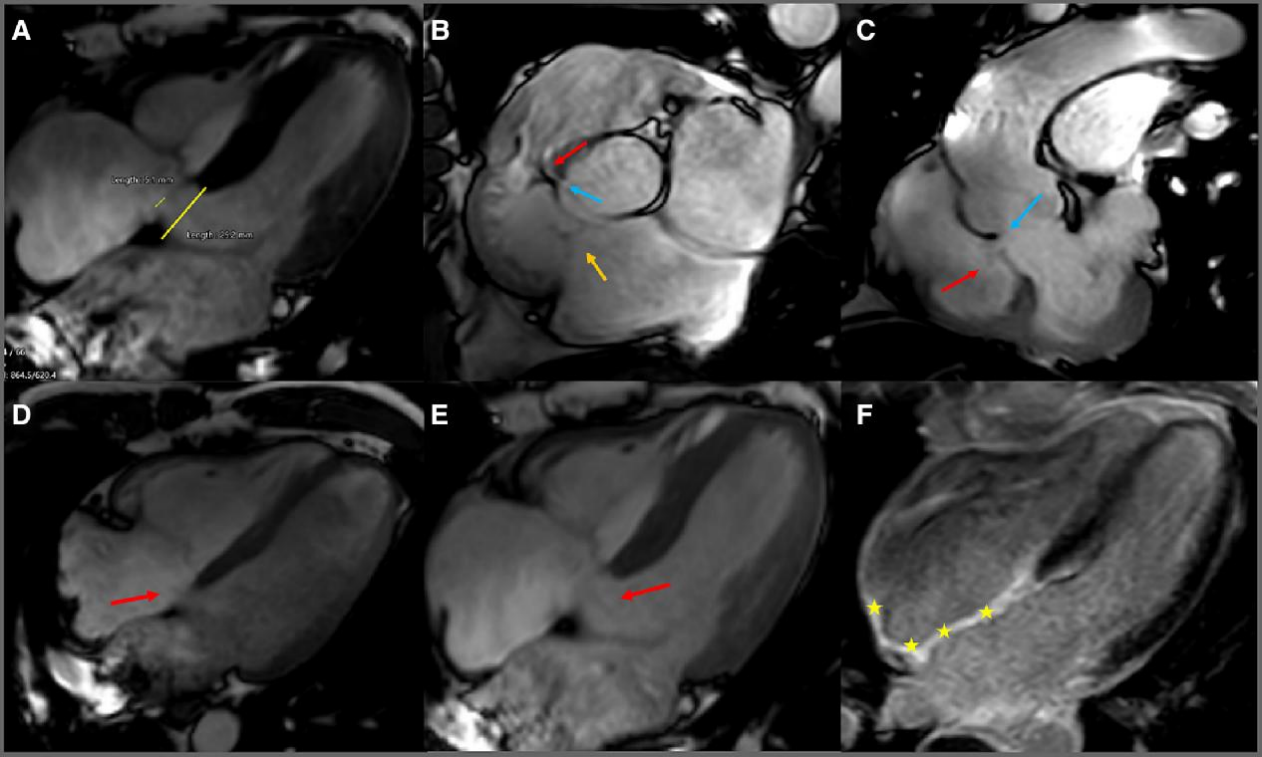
(*A*) Contrast magnetic resonance imaging showing apical displacement of septal leaflet of the tricuspid by 12.5 mm/m^2^—Ebstein’s anomaly (broad line)—and a 5.1 mm communication (thin line) between the LV and the RA, Gerbode defect. (*B*) Modified short-axis cine of the aortic valve—TV (yellow arrow), defect (blue arrow), flow acceleration (red arrow). (*C*) Cine left ventricular outflow tract sequence showing the defect (blue arrow) and the flow acceleration (red arrow)—left-to-right shunt. (*D*) Left-to-right shunt (arrow). (*E*) Right-to-left shunt (arrow). (*F*) LGE at the level of the ight atrium (star). CMR, contrast magnetic resonance; LGE, late gadolinium enhancement; LV, left ventricle; LVOT, left ventricular outflow tract; RA, right atrium; RV, right ventricle; TV, tricuspid valve.
